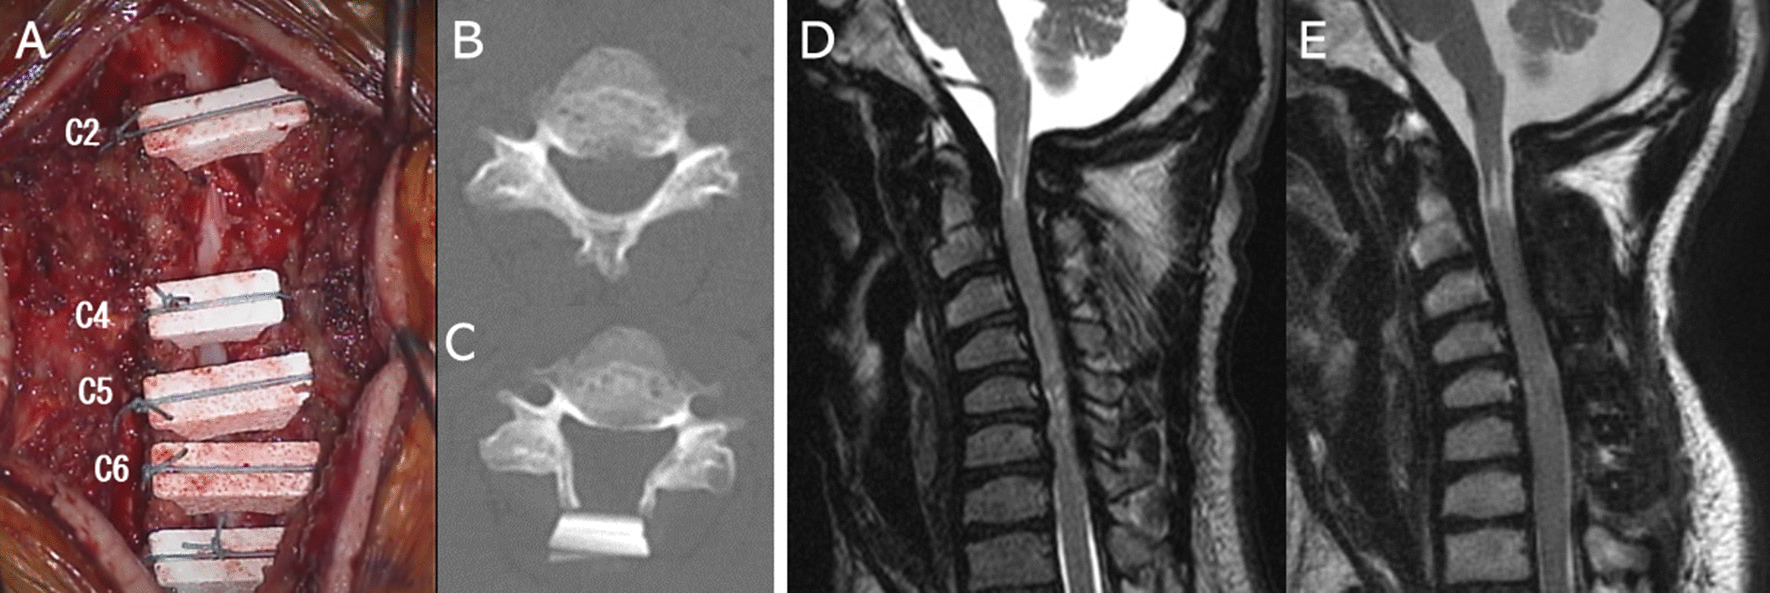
A 37-year-old male patient with MPS type II. Double door laminoplasty combined with C1 posterior arch resection and partial resection of the foramen magnum dorsal wall. A HA spacers were placed and ligated between the opened laminae. B Axial CT image of the lamina before operation. C Opened laminar and HA spacer (same level as B). D T2WI MR image of the cervical spine before operation. E T2WI MR image of the same patient at 6 months after surgery. Note that the cervical spinal canal was expanded. The high intensity area at the C1/2 level became more evident and that at the C5/6 level disappeared after surgery. * MPS* mucopolysaccharidoses, *HA* hydroxyapatite, *CT* computed tomography, *MR* magnetic resonance
